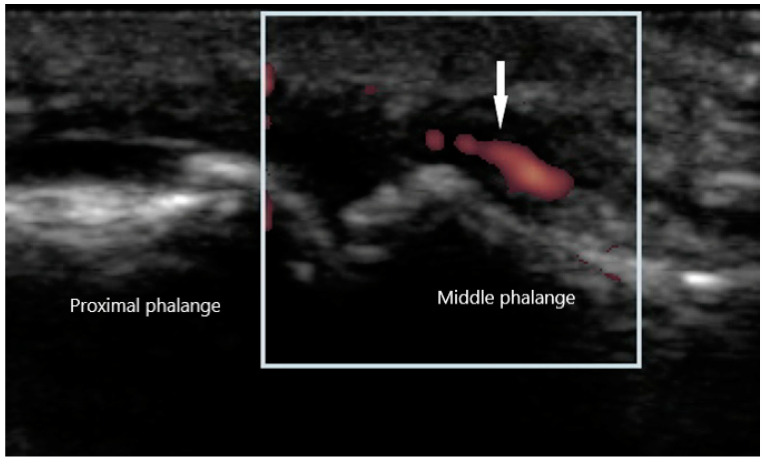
Synovitis of proximal interphalangeal joint with power Doppler signal (arrow).
